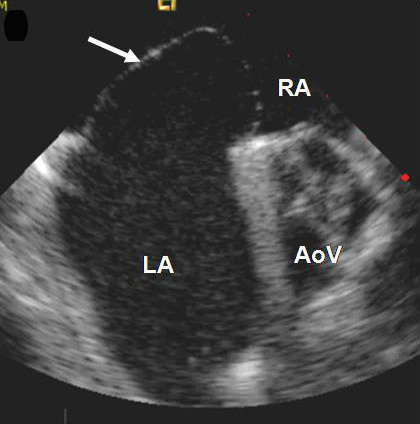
Image obtained using intracardiac echocardiography (ICE): Demonstrating a large interatrial aneurysm (arrow) billowing into the right atrium (RA) with an excursion of over 22 mm.
